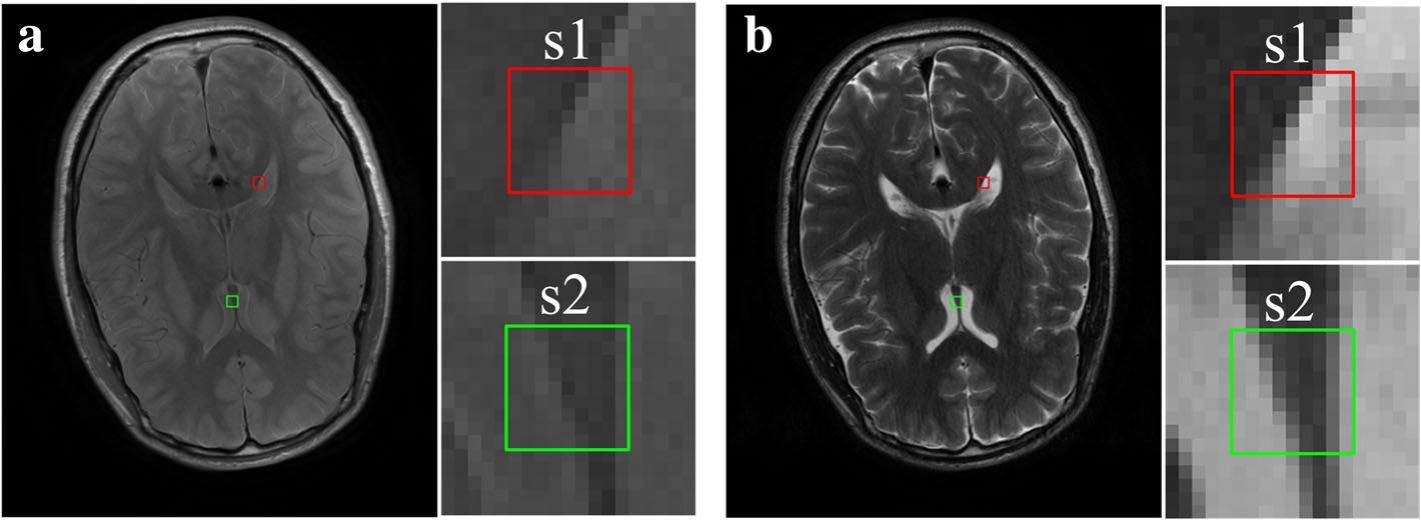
Regression weights within local regions of T1-weighted and T2-weighted MRI images. a is the T1-weighted image; b is the T2-weighted image. Two pairs of image region of size 9 × 9 (enclosed in wireframes, marked as S1 and S2) are extracted from (a) and (b). Note: The data are acquired on a 3 T SIMENS scanner
